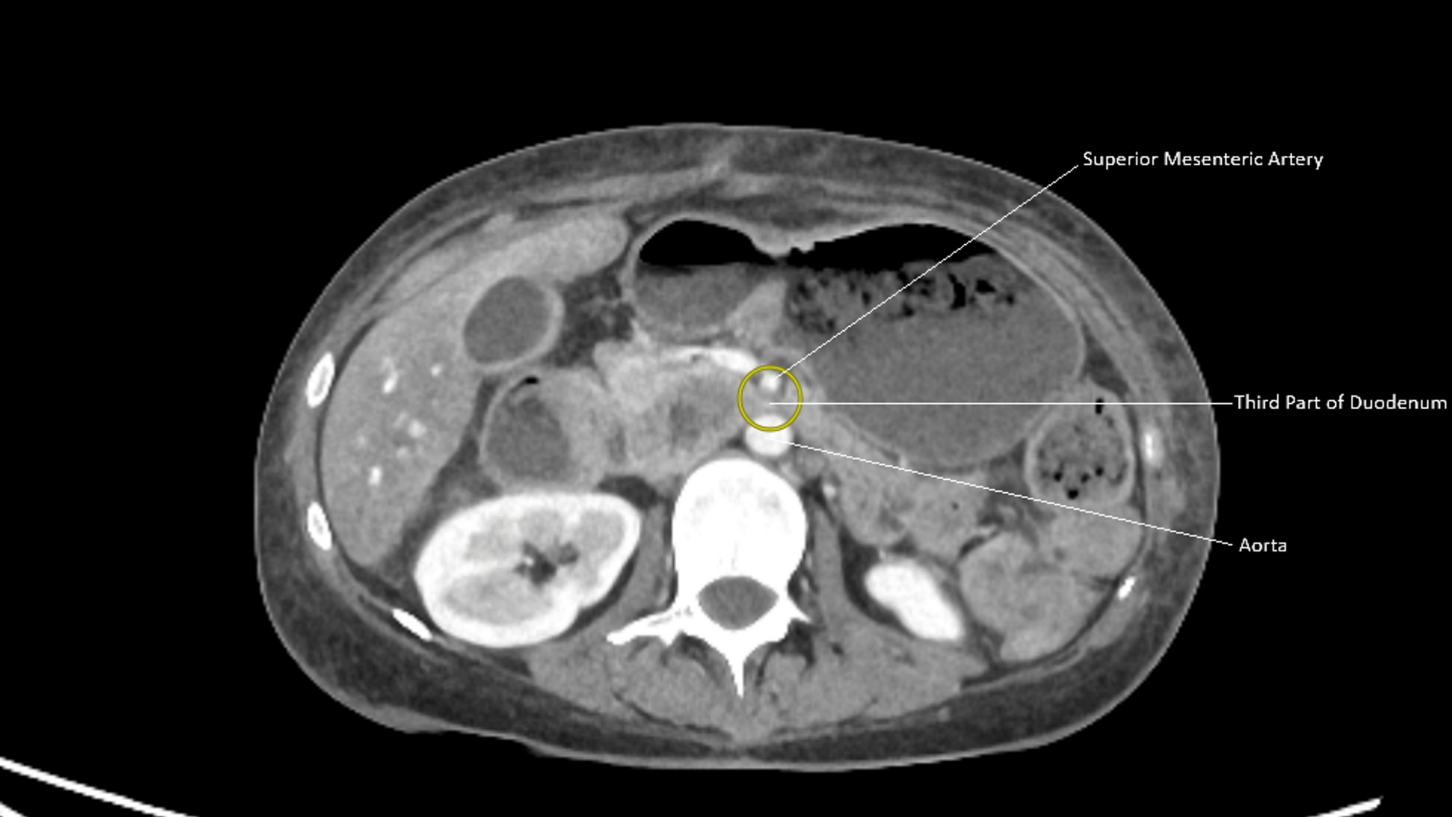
Axial CT showing compression of the third part of the duodenum between the superior mesenteric artery (SMA) and aorta, consistent with SMA syndrome.
